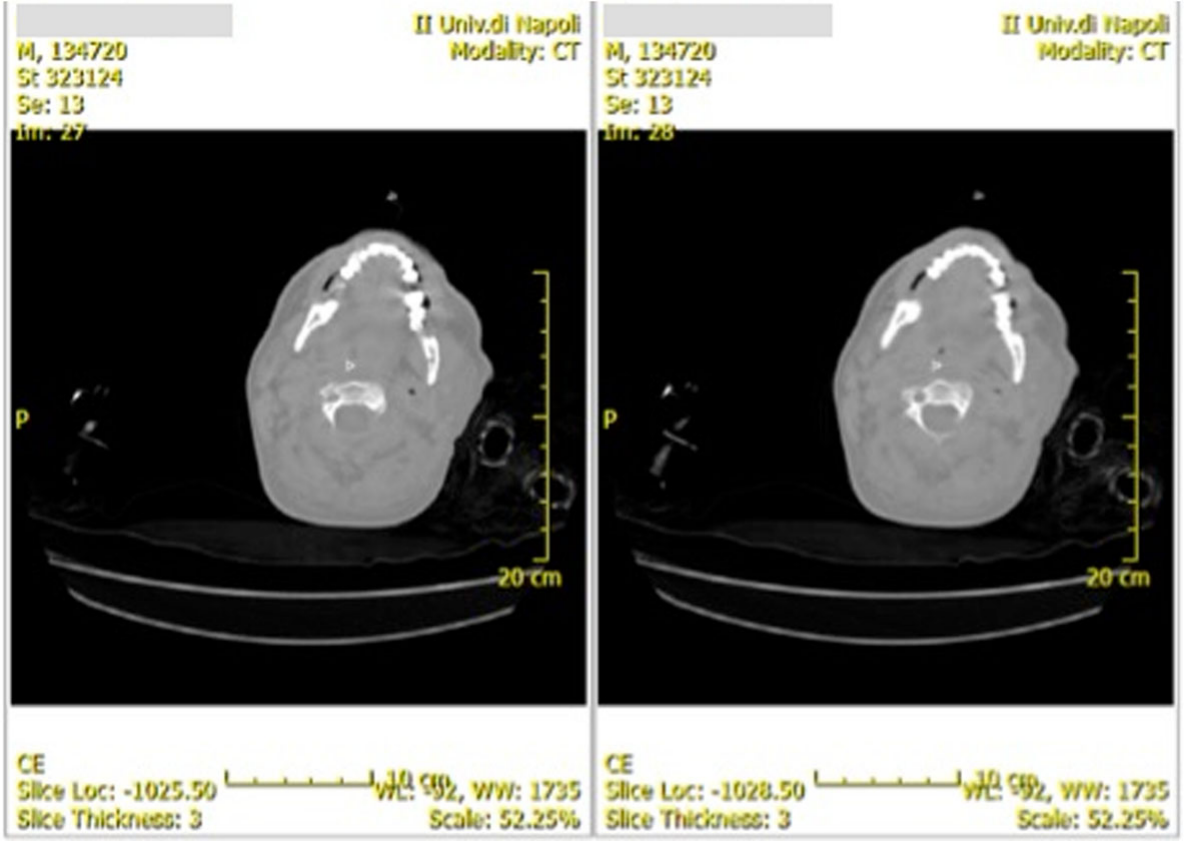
Thoracic computed tomography scan
